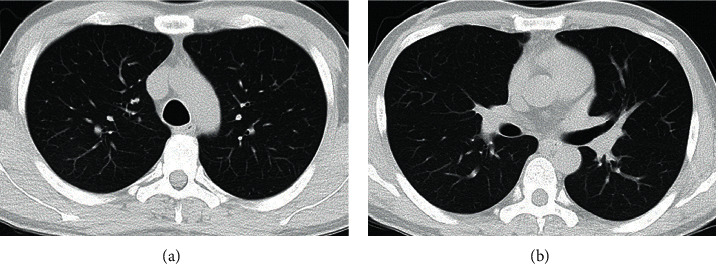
Postoperative chest computed tomography (CT) radiological findings. A chest CT in the lung window setting showed no consolidation or ground-glass opacity.
